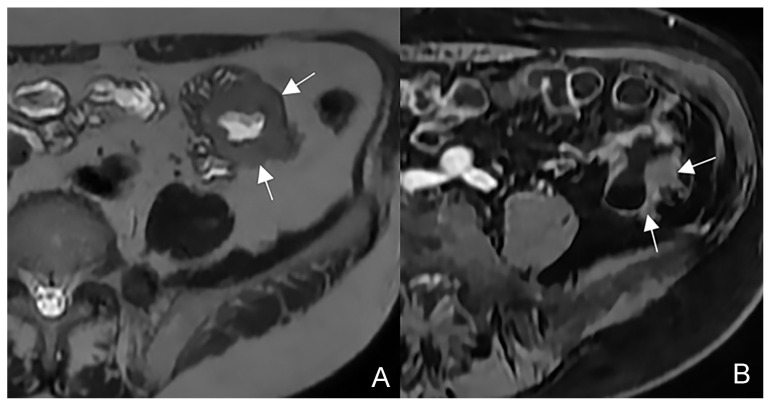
Example of GIST in an old man with abdominal discomfort and a palpable mass. (A) Axial T2-weighted and (B) axial T1-weighted after gadolinium injection images show an asymmetrical mass-like thickening of a jejunal loop (arrows) with aneurysmal pattern.
